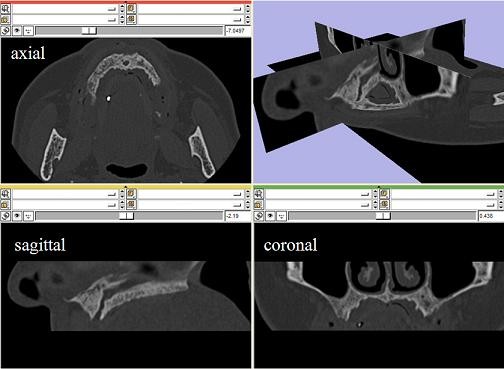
CT data. Maxilla CT data in the axial, sagittal and coronal planes and a fully 3D vision
